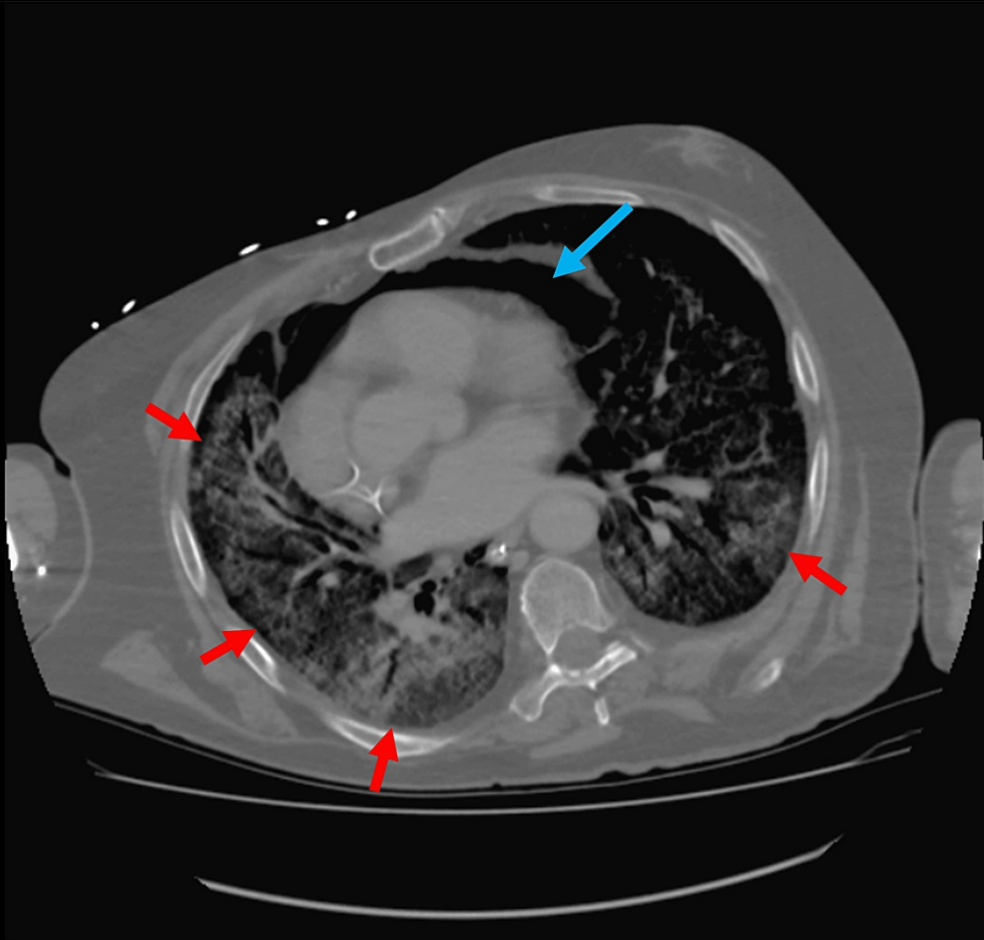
CT chest with a marked progression of bilateral opacities (red arrows) and development of pneumopericardium (blue arrow).
